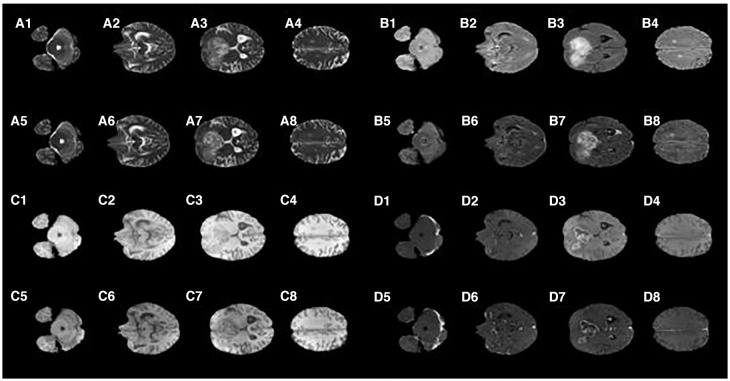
Synthetic MRI compared with patient MR images. (A1–A4), synthetic T2; (A5–A8), patient T2; (B1–B4), synthetic Flair; (B5–B8), patient Flair; (C1–C4), synthetic T1; (C5–C8), patient T1; (D1–D4), synthetic T1CE; (D5–D8), patient T1CE.
